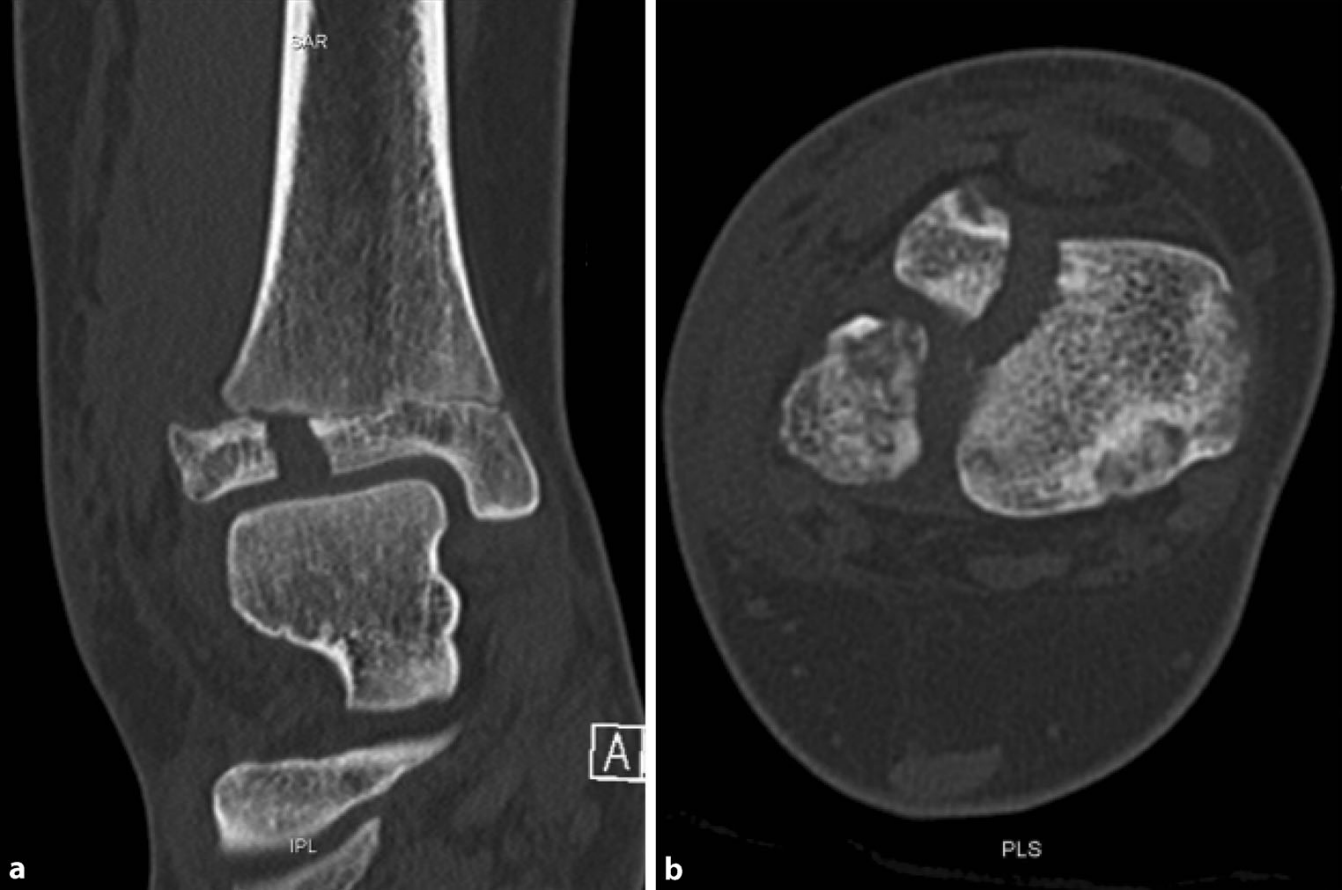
CT scan, coronal (a) and axial plane (b) of a Tillaux fracture in a 14-year-old patient
